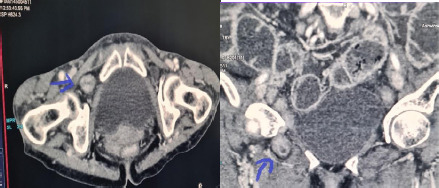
The axial and coronal images of the CT scan showing right sided obturator hernia containing a bowel loop (marked by an arrow) causing an obstruction.
